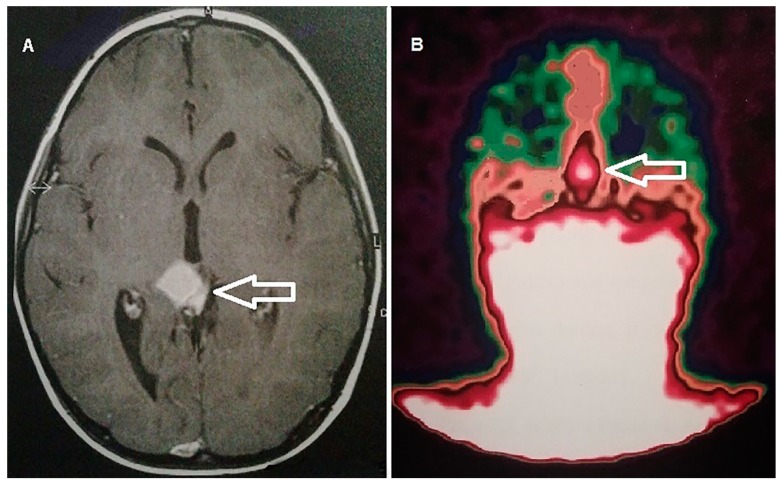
An eight year-old-male patient with sudden onset of nausea/vomiting, headache, and diplopia. An MRI scan (A) disclosed a large tumor (white bordered arrow) in the pineal region of the brain. Somatostatin receptor scintigraphy with [^111^In] pentetreotide (B) demonstrated intense tracer uptake corresponding to the brain lesion (white bordered arrow), consistent with high somatostatin receptor (subtype 2) expression. The patient underwent surgery. The final diagnosis was pineoblastoma.
